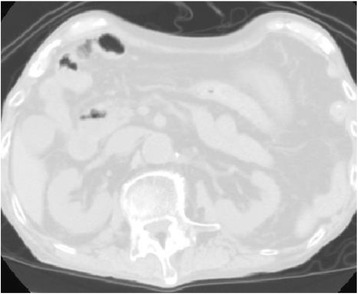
Computed tomography after hyperbaric oxygen treatment shows no gas located in the abdominal wall, bowel wall, or mesentery
